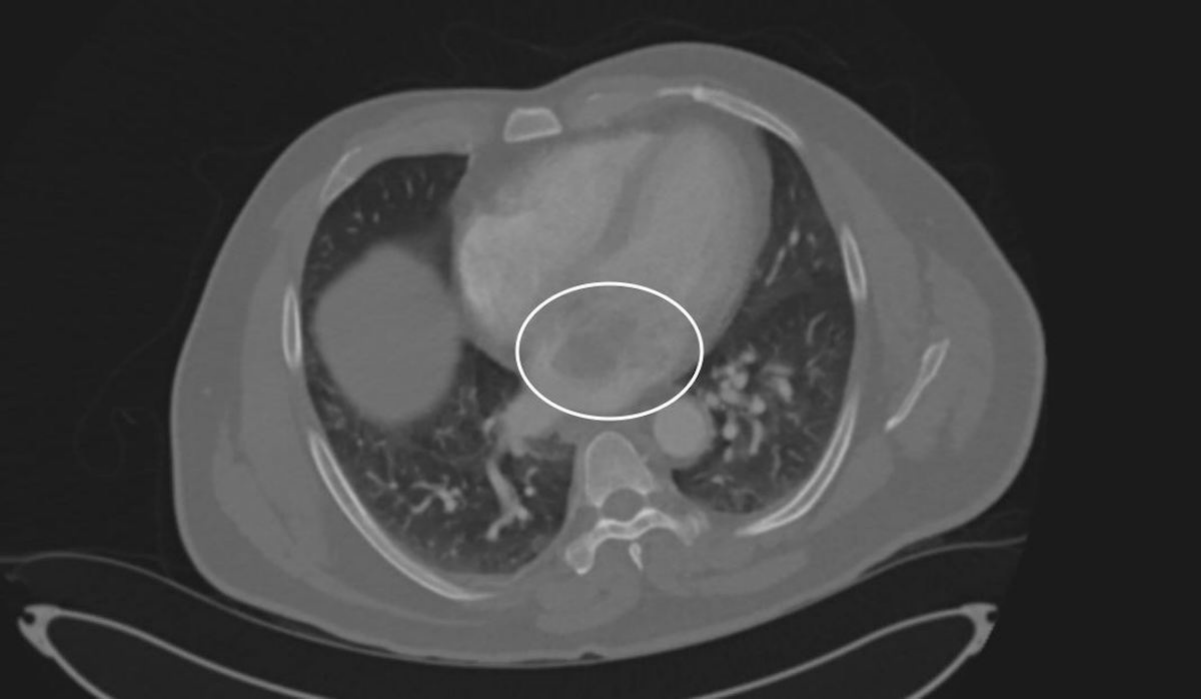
Computed tomography with contrast of chest (Transverse view) demonstrating a soft tissue mass in the left atrium.
